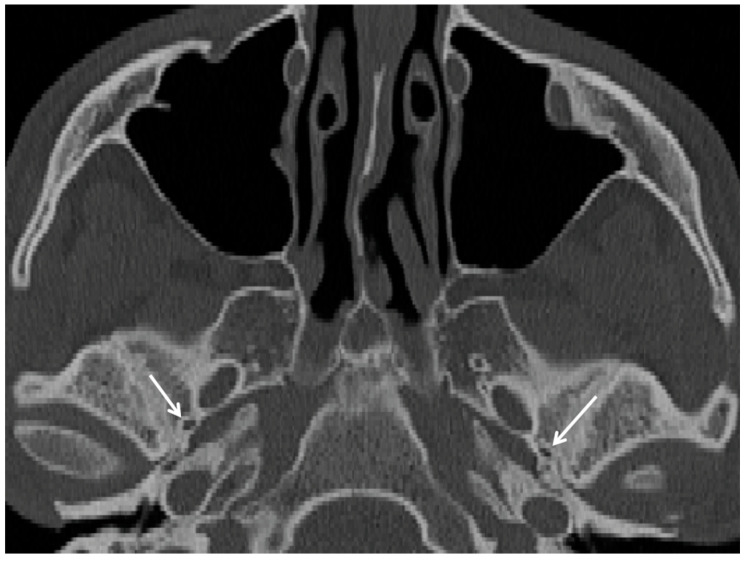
Tranverse CT scan of an individual with the bilateral canaliculus innominatus (white arrows).
